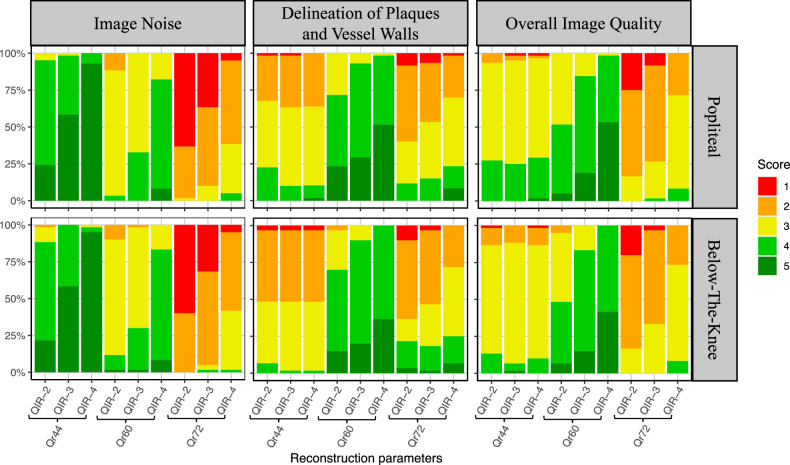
Qualitative image analyses of image noise, delineation of plaques and vessel walls, and overall CTA image quality at the popliteal (Pop) and below-the-knee (BTK) levels
